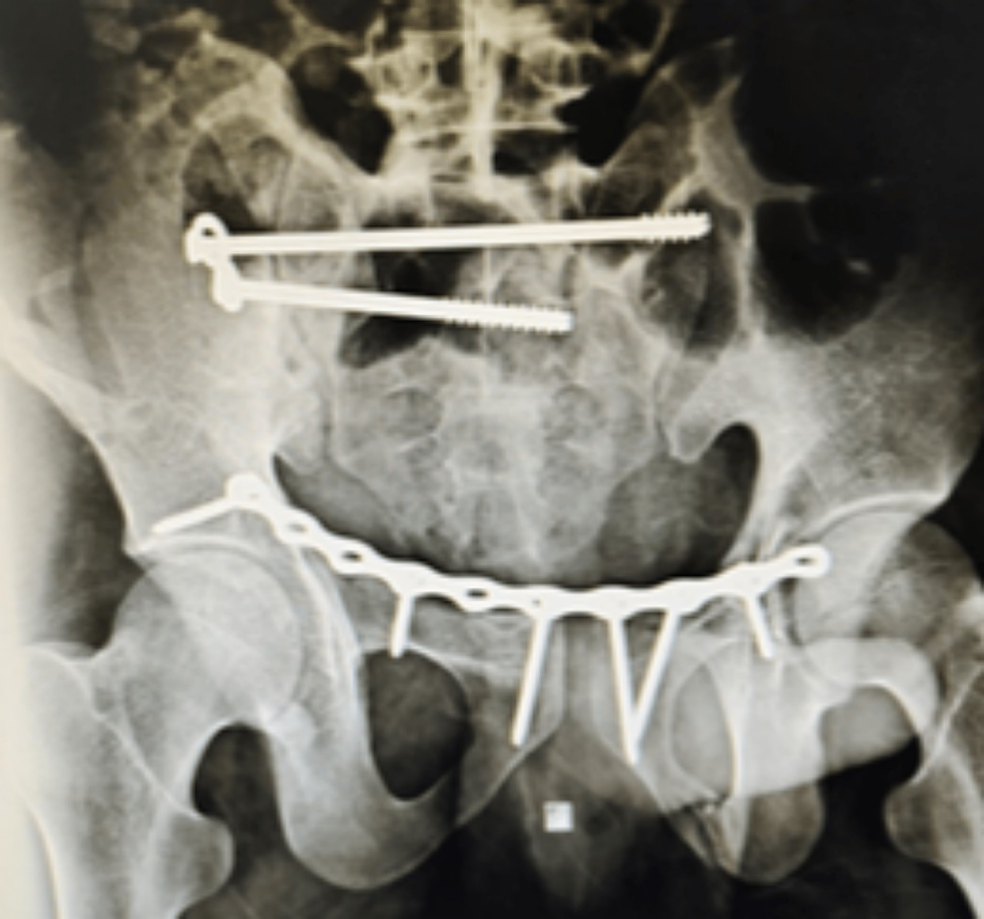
Post-operative X-ray showing pelvic outlet
